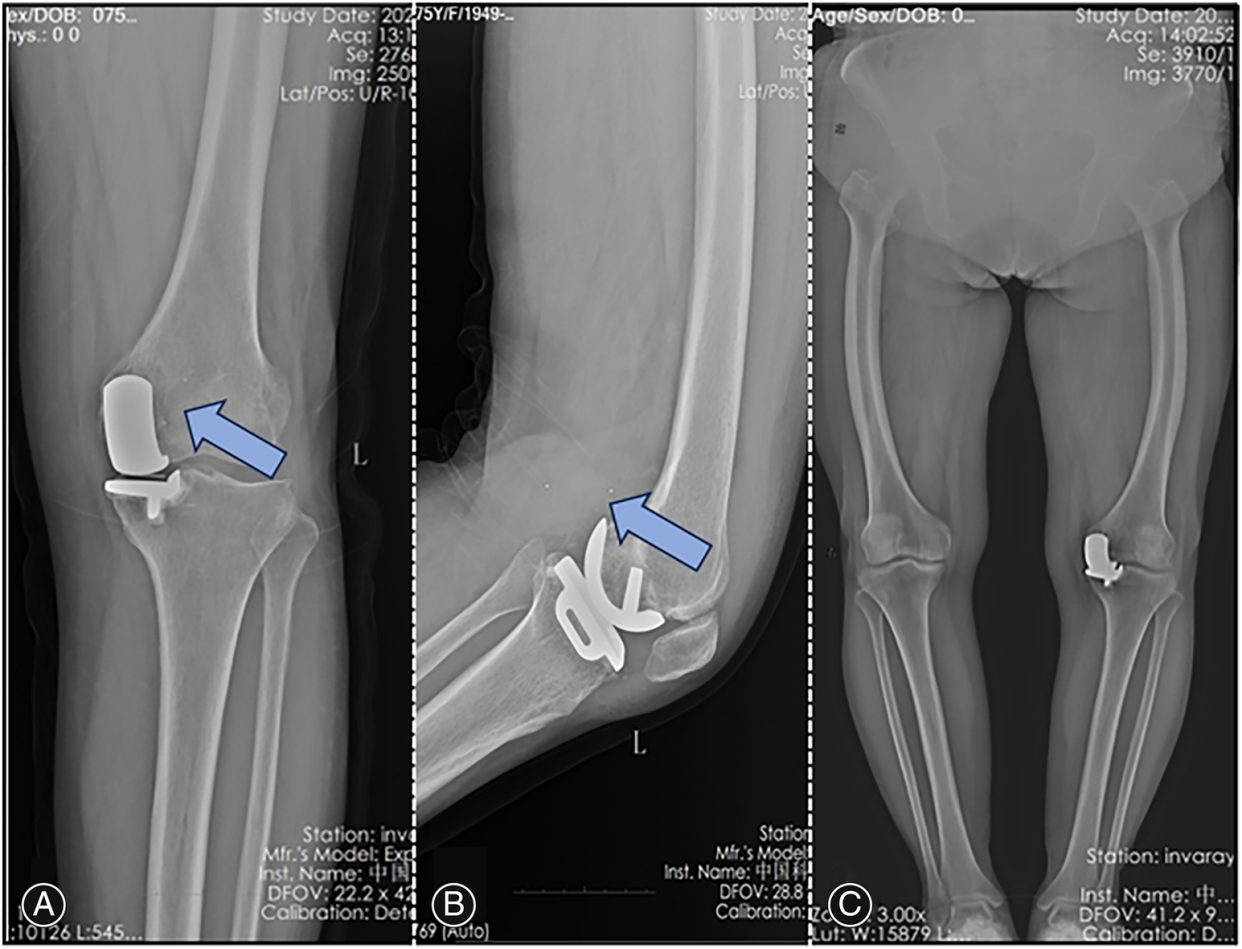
Preoperative radiographs demonstrating that medial unicompartmental knee arthroplasty with mobile bearing fracture and posteromedial dislocation. (A) AP, (B) lateral, and (C) full‐length weight‐bearing of the lower extremities. Blue arrows indicate broken bearing fragments.
